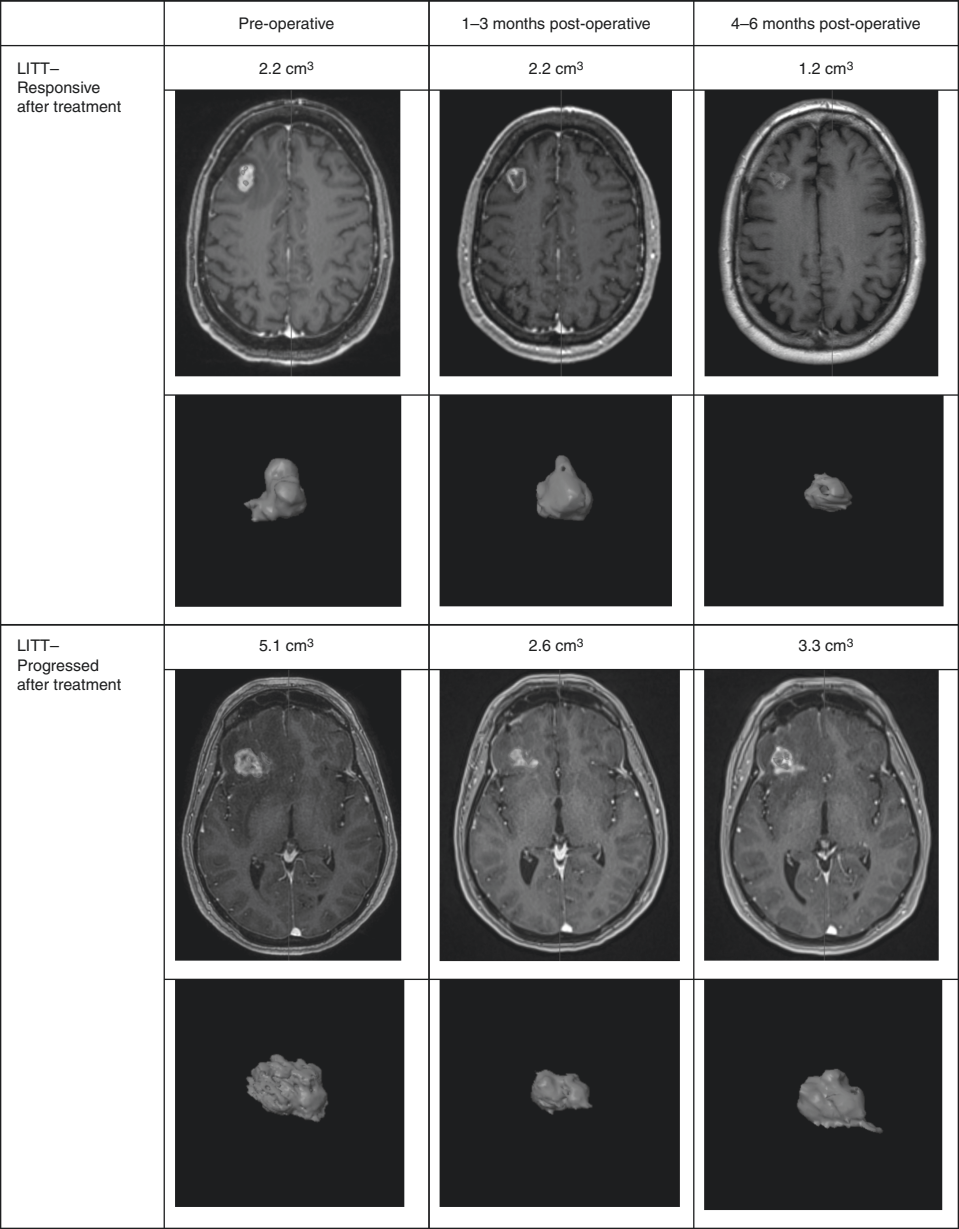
Representative imaging evolution of LITT-treated lesions. The upper images were obtained from T1 post-contrast MRI sequences. The lower images depict volumetric models of the mapped lesions. LITT, laser interstitial thermal therapy.
